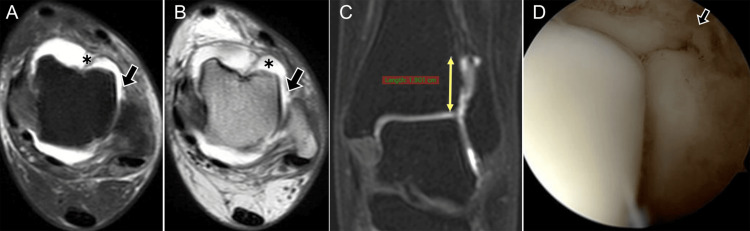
Combined syndesmotic and ATFL injury. (A) Axial STIR MRI (short tau inversion recovery) and (B) post-contrast T1 spin-echo MRI show a full-thickness ATFL tear (arrow) with contrast leakage (*); (C) coronal T1 fat-suppressed MRI shows contrast extending 1.9 cm above the syndesmosis (yellow line); (D) arthroscopy confirms ATFL rupture (arrow). ATFL: anterior talofibular ligament Images belong to the authors; patient consent was obtained.
